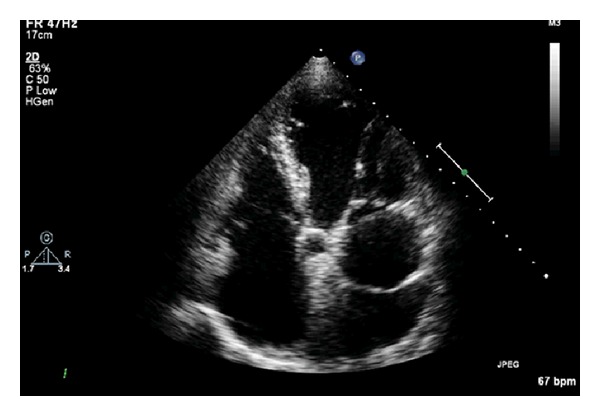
Two-dimensional echocardiographic view (apical four chamber view) showing cor triatriatum sinistrum.
